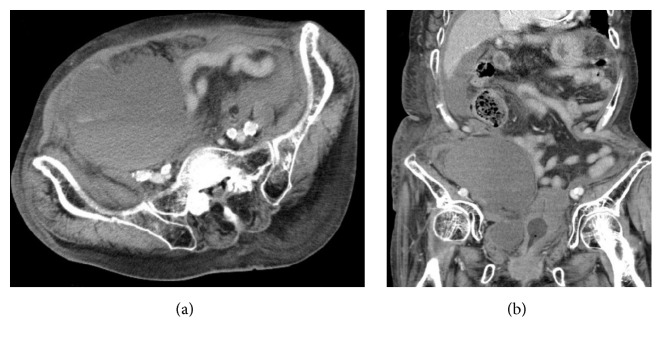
Abdominal computed tomography scans with intravenous contrast showing axial and coronal views. A massive preperitoneal hematoma is present beneath the right hypogastric vessels with hemoperitoneum.
